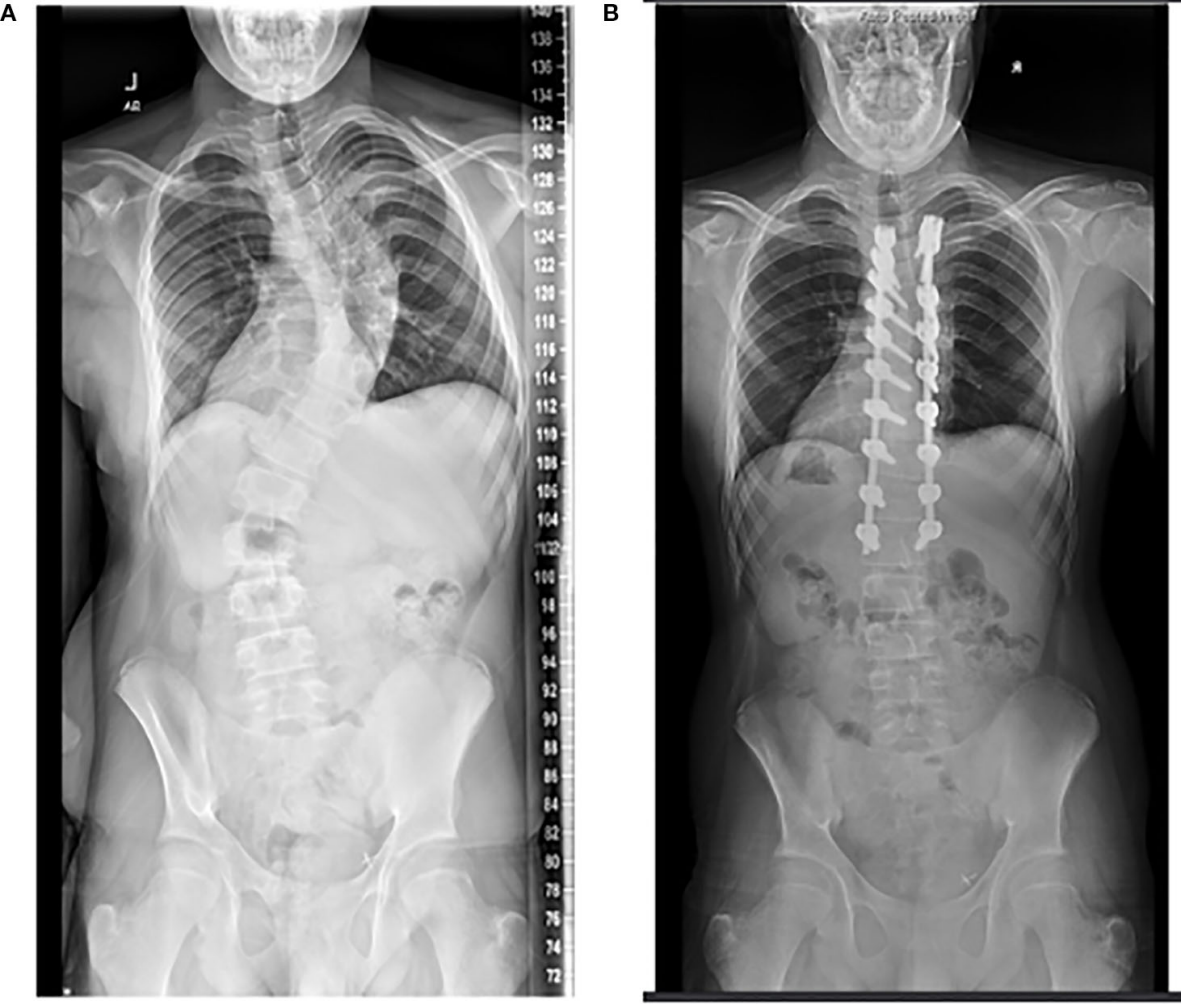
Radiographs of a 12-year-old with significant genu valgum (A). She underwent left sided corrective osteotomies and intramedullary nailing of both the femur and tibia at 13 years old. She then underwent right sided deformity corrections at the age of 14. Radiograph of the corrected lower limb alignment at 15 years old (B).
